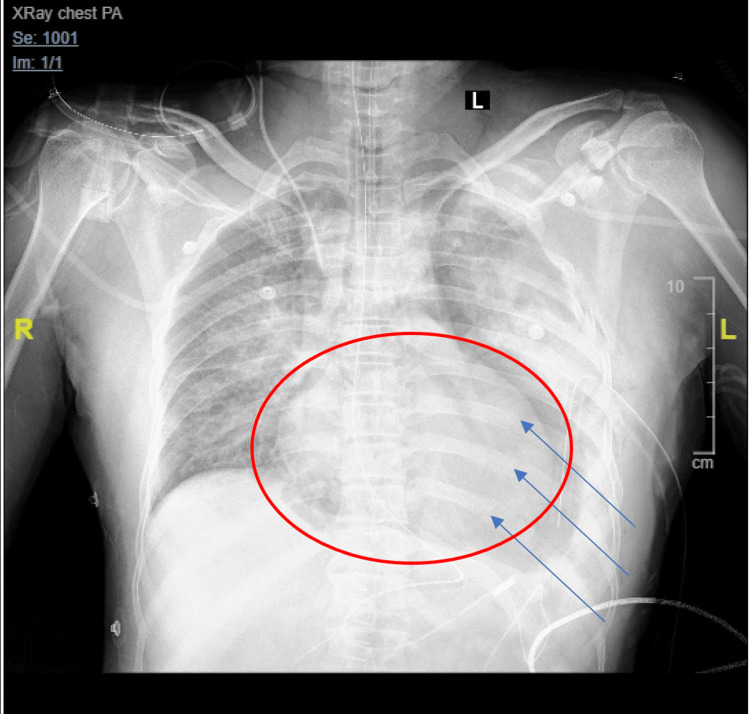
An anterior-posterior chest radiograph shows a left diaphragmatic hernia with protrusion of internal abdominal organs into the chest, visualized by the pericardial lucency (red circle) that represents the herniated bowel and multiple left-sided rib fractures (blue arrows) with underlying hemothorax.
